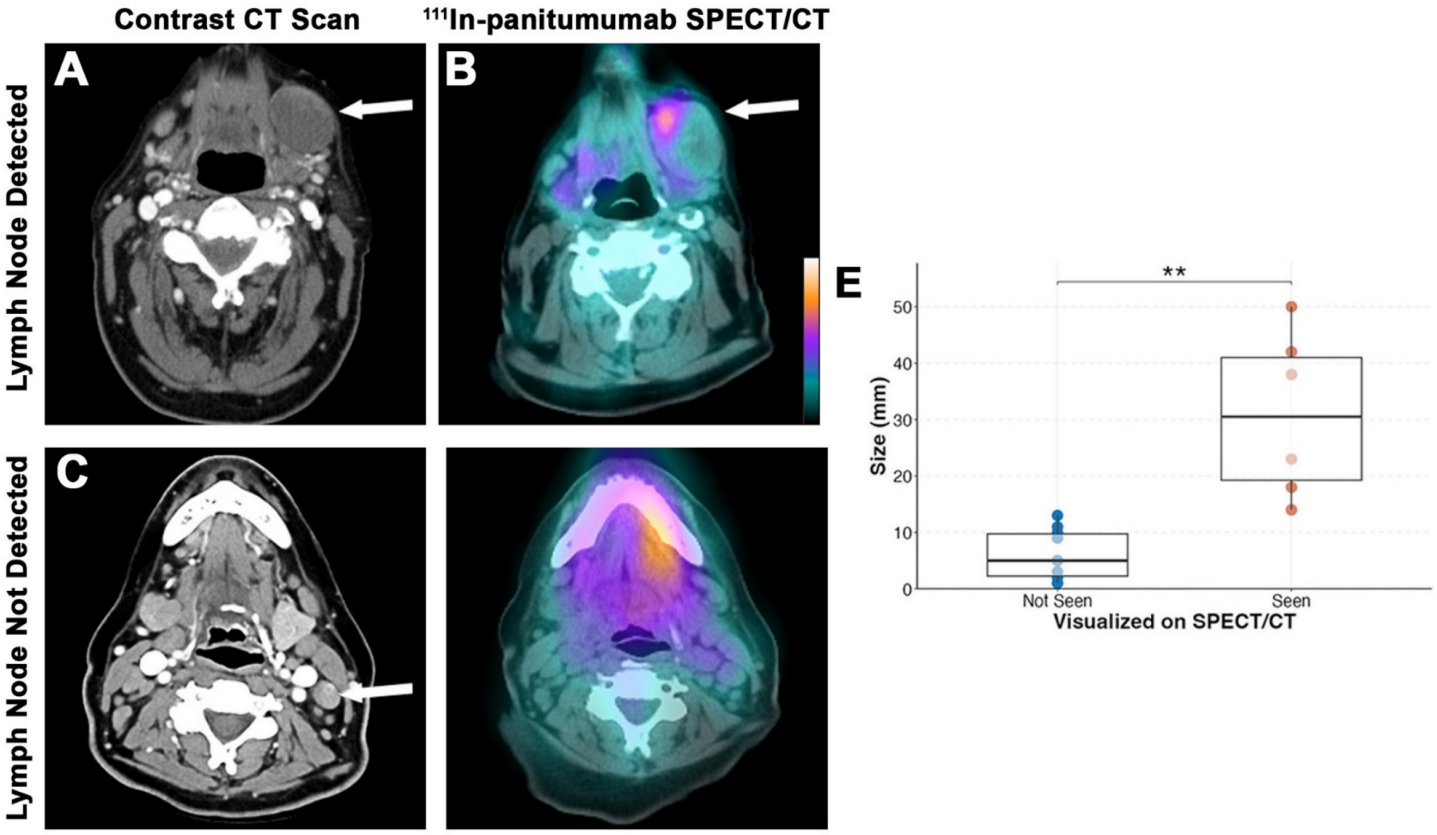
SPECT/CT detection of lymph nodes. Representative preoperative contrast-enhanced CT scan (A) and [111In]panitumumab scan (B) where a large metastatic lymph node measuring 5 cm in diameter on final pathology had clear localization of the radiotracer. Representative preoperative CT scan (C) from a different patient showing a smaller suspicious lymph node with calcification that was not definitively localized on [111In]panitumumab SPECT/CT (D). Size breakdown (E) of positive lymph nodes seen compared to neck location (F).
